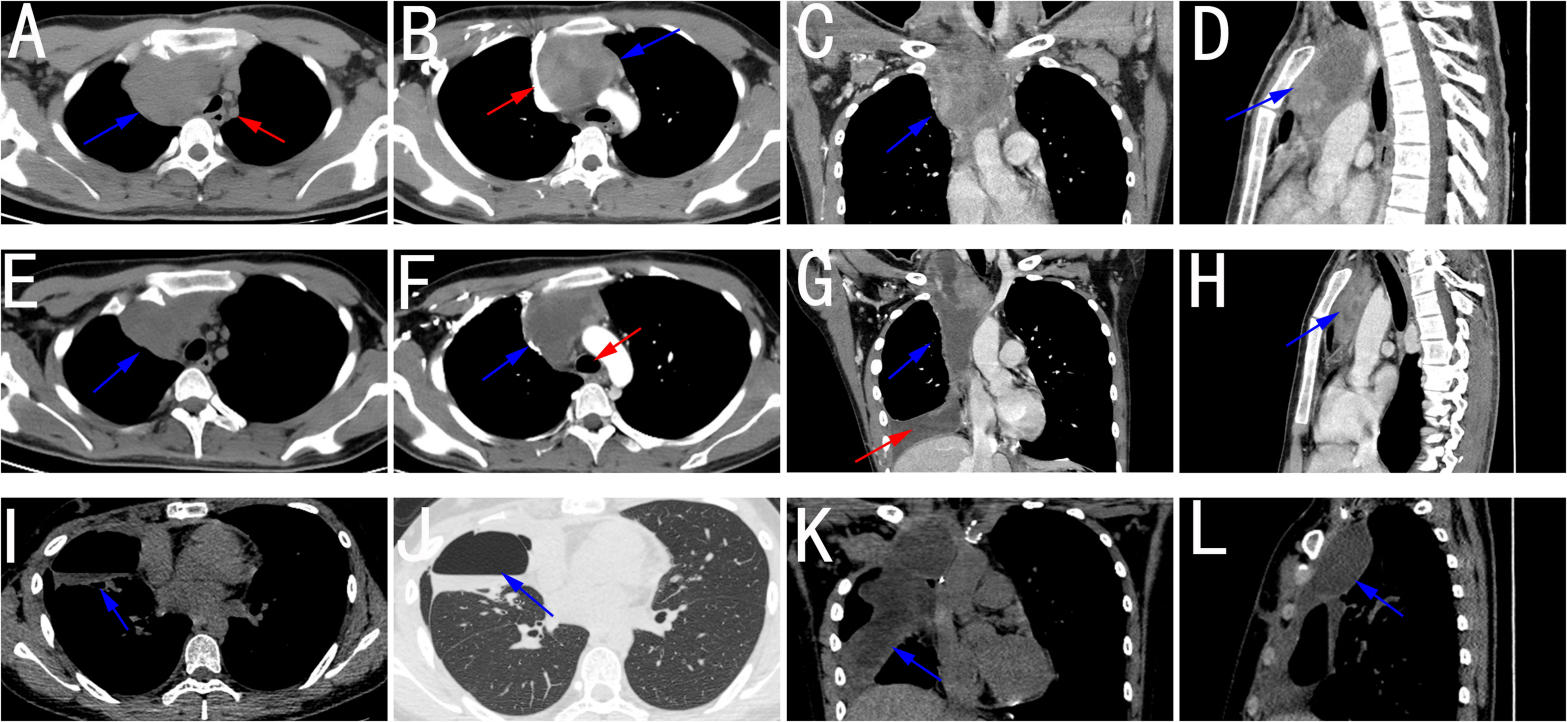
(A) Chest plain CT scan showing a mass with an irregularly round shape (blue arrow) that compressed the adjacent structure (red arrow) in the superior mediastinum, including the trachea and vessels. (B) Contrast-enhanced chest CT scan showing that the enhancement of the lesion was uneven and obvious (blue arrow), with a compressed and irregularly narrow superior vena cava (red arrow). (C, D) Coronal and sagittal reconstruction showing the position of the cystic and solid mass (blue arrow) in the superior mediastinum. (E, F) After a four-month course of combined chemotherapy, the chest CT scan and contrast-enhanced chest CT scan showed shrinkage of the mass and decreased compression of surrounding structures, such as the trachea (red arrow). (G, H) Coronal and sagittal reconstruction also showing a reduction in the mass volume and a decrease in the solid component (blue arrow), accompanied by pleural effusion (red arrow). (I, J) CT scan two months after surgery showing hydropneumothorax in the thoracic cavity (blue arrow). (K, L) CT scan two months after surgery showing encapsulating cystic fluid accumulation near the surgical area (blue arrow).
